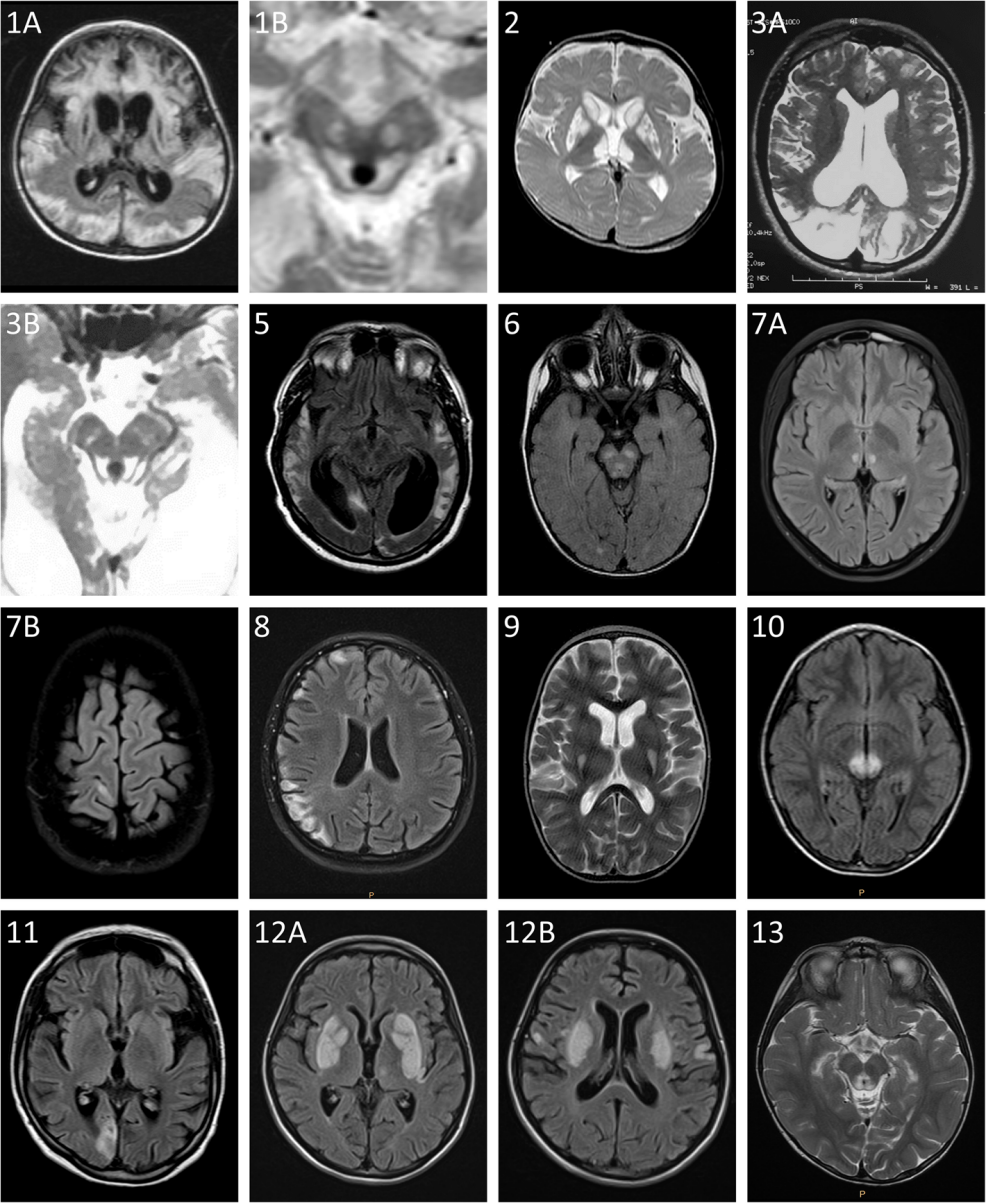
MRI of the brain in P1–P3 and P5–P12 with complex I deficiency. Signal changes in basal ganglia or brainstem characteristic for Leigh syndrome are present in patients P2, P6, P9, P10 and P13. Signal changes in the cortex and white matter of the hemispheres or cerebellum with stroke-like lesion are present in patients P5, P8, P11, and the combination of both changes are visible in patients P1, P3, P7 and P12. Moderate to severe periventricular atrophy was found in patients P1, P3 and P5.
